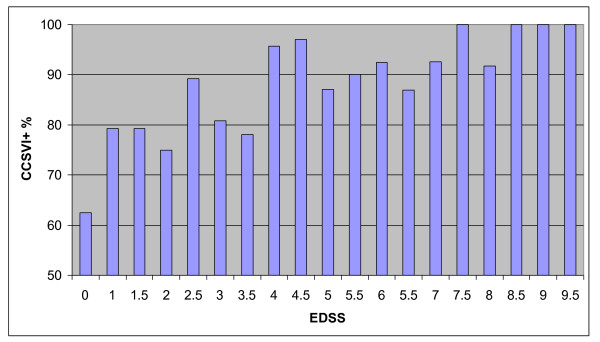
Percentages of CCSVI-positive (CCSVI+) patients according to their EDSS score at the time of Doppler evaluation.
